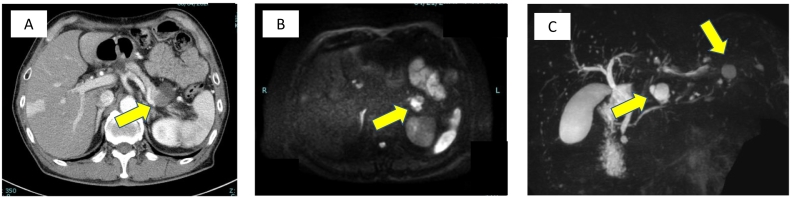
Imaging studies at the time of pancreatic tail cancer diagnosis. A: Contrast-enhanced computed tomography. A solid lesion with a cystic component in the pancreatic tail is observed. B: MRI (diffusion-emphasizing image). A high signal is noted in the pancreatic tail. C: Magnetic resonance cholangiopancreatography: Cystic lesions are observed in the pancreatic body and tail.
